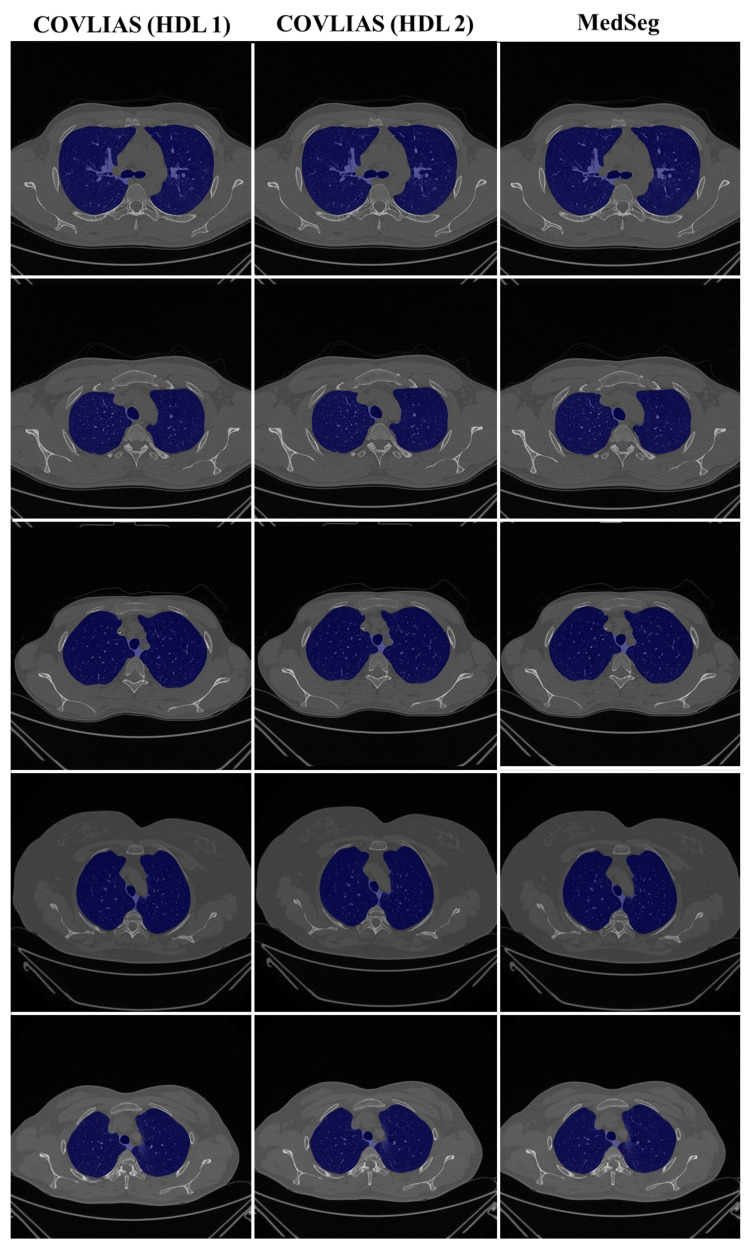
COVLIAS vs. MedSeg: Segmented mask (blue) on the Control CT lung image (small lung).
